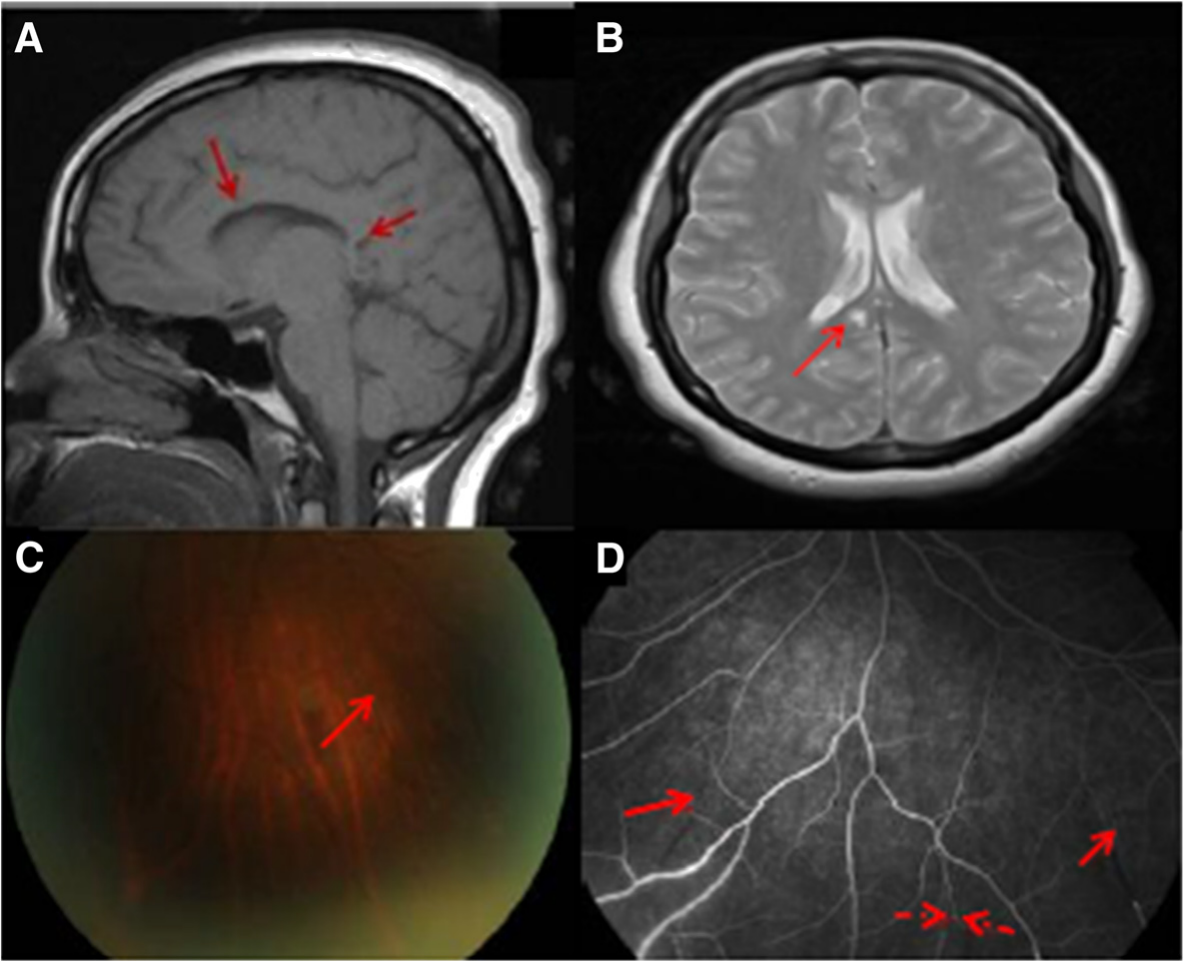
SS findings on MRI brain (A, B), fundus photographs (C) and fundus angiography (D). A. T1-weighted MRI brain showing punched out lesions in the corpus callosum (arrowed) typical of Susac’s syndrome. B. Axial T2- weighted MRI brain showing typical “snow ball” appearance of inflammation in the posterior corpus callosum (arrow) C. Colour fundus photograph showing branch retinal artery occlusion (arrowed) in the peripheral fundus (OS). D. Fundus fluorescein angiographic image showing the same lesion (broken arrow) and additional occlusions not previously recognised on clinical examination alone (unbroken arrows).
